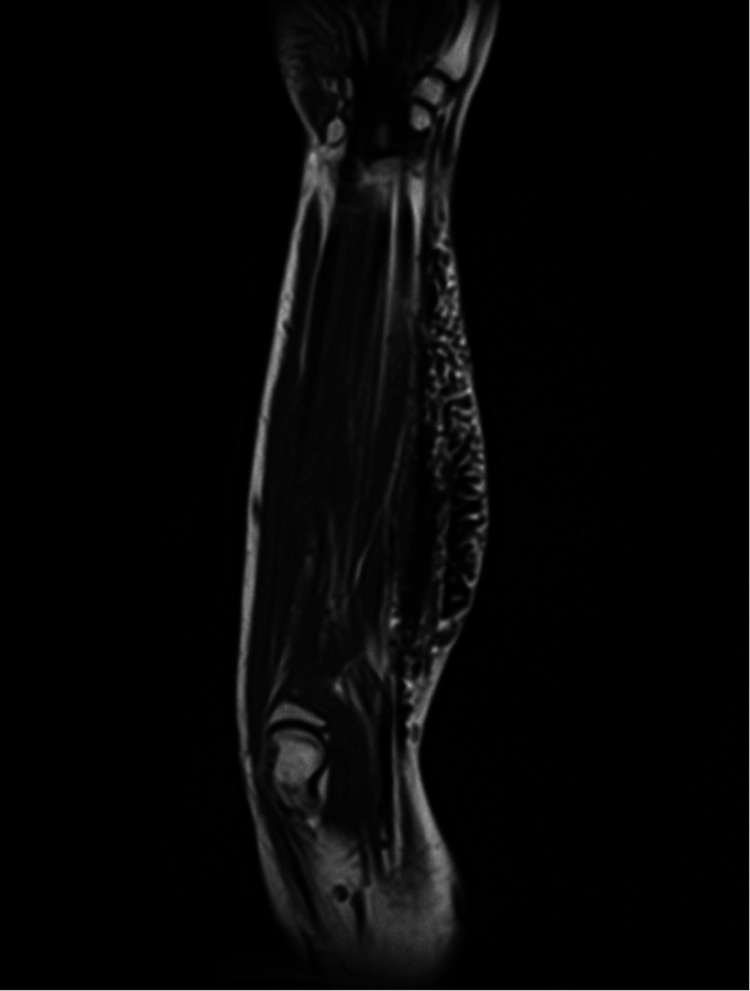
MRI of right forearm MRI of the right forearm shows subcutaneous emphysema and changes of cellulitis involving the anterolateral aspect of the forearm with extensive edema signals in proximal intermuscular facial planes without any definitive evidence of osseous or muscular involvement.
